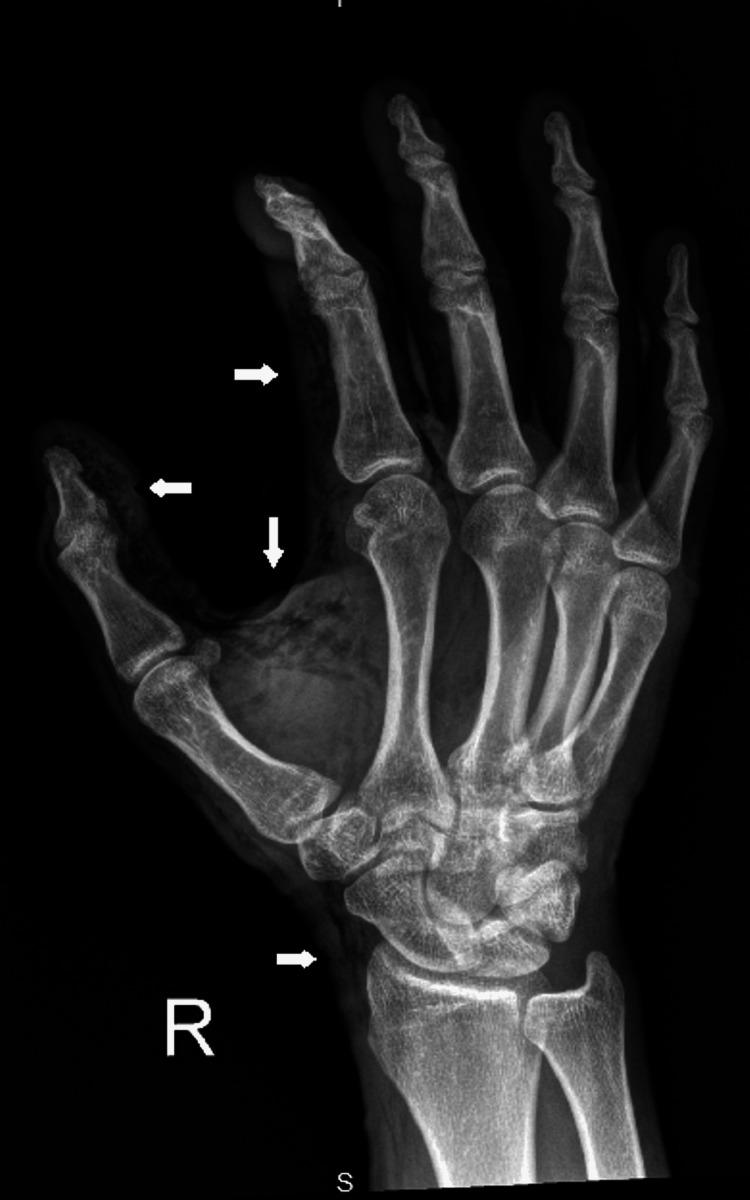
An X-ray showing extensive subcutaneous edema and emphysema (white arrows) within the right thumb, index finger, and middle finger, which extends to the wrist and palmar soft tissue.
